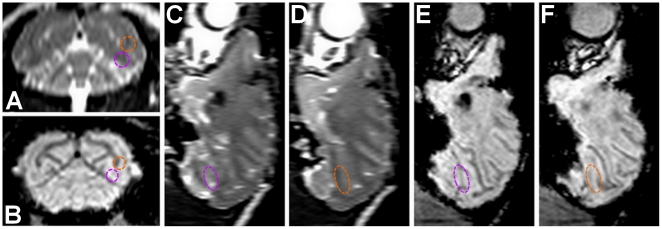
Damage assessment. (A,C,D) 3D T2-weighted sequence. Edemas should appear brighter in these images. (B,E,F) 3D Susceptibility-Weighted Image (SWI) sequence was applied. Hemorrhages, as well as large vessels should appear in black in these images. (A,B) Same reconstructed coronal slice as shown in figure 1. The two opening sites are circled with the corresponding colors. There is no difference between the two hemispheres. (C,D,E,F) Corresponding reconstructed sagittal slices for the two opening sites. No oedemas or hemorrhages are visible.
